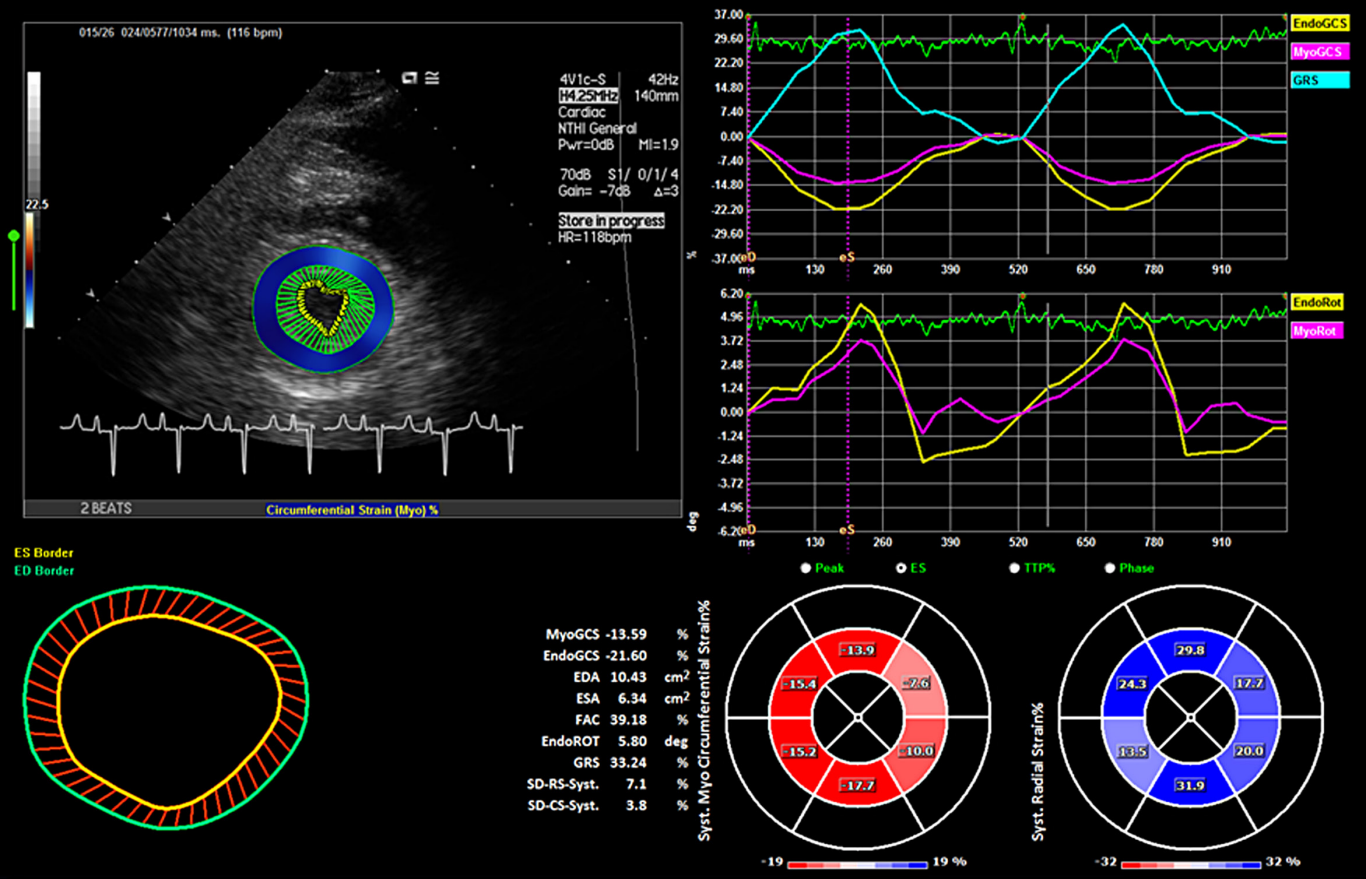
Analytical interface for unenhanced imaging in the parasternal short axis view, showing the EDA, ESA, FAC results (lower left panel) and the EGCS, MGCS, GRS and ER (upper right panels). Video 1 shows the corresponding clip.
